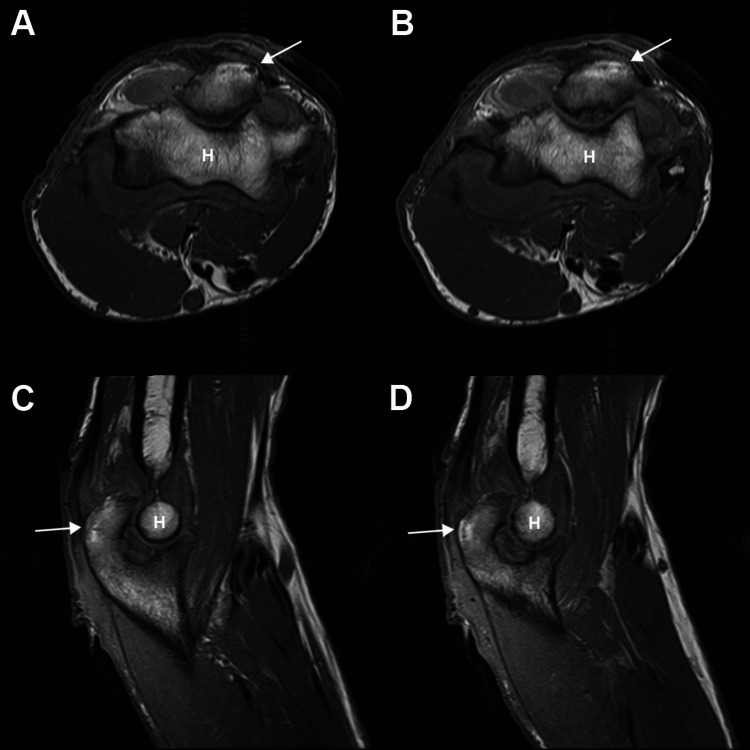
MR Images of Patient's Elbow Magnetic resonance (MR) T1-weighted images of our patient’s elbow in axial (A and B) and sagittal (C and D) views. Arrows point to increased signal in the olecranon, suggestive of early osteomyelitis. “H” indicates the condylar region of the humerus. Arthritis of the elbow joint, primarily of the olecranon articular surface, is also seen in the sagittal views.
